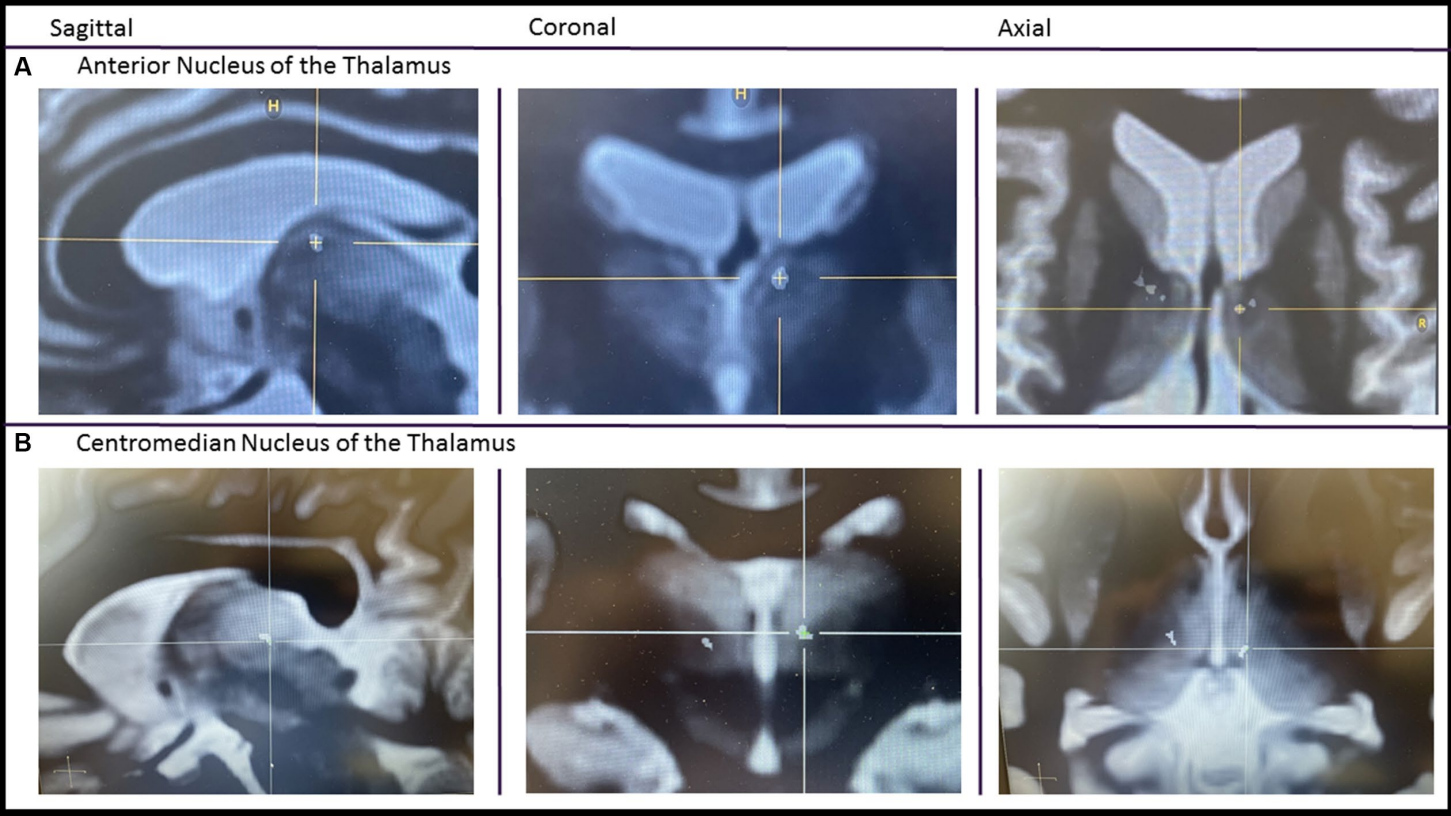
Imaging of thalamic RNS. A pre-op FGATIR MRI used for targeting is fused with a post-op volumetric CT. After fusion, the CT scan is made translucent except for the contacts. Imaging centered on the most internal contact of the left anterior thalamic lead (A) and centromedian lead (B).
